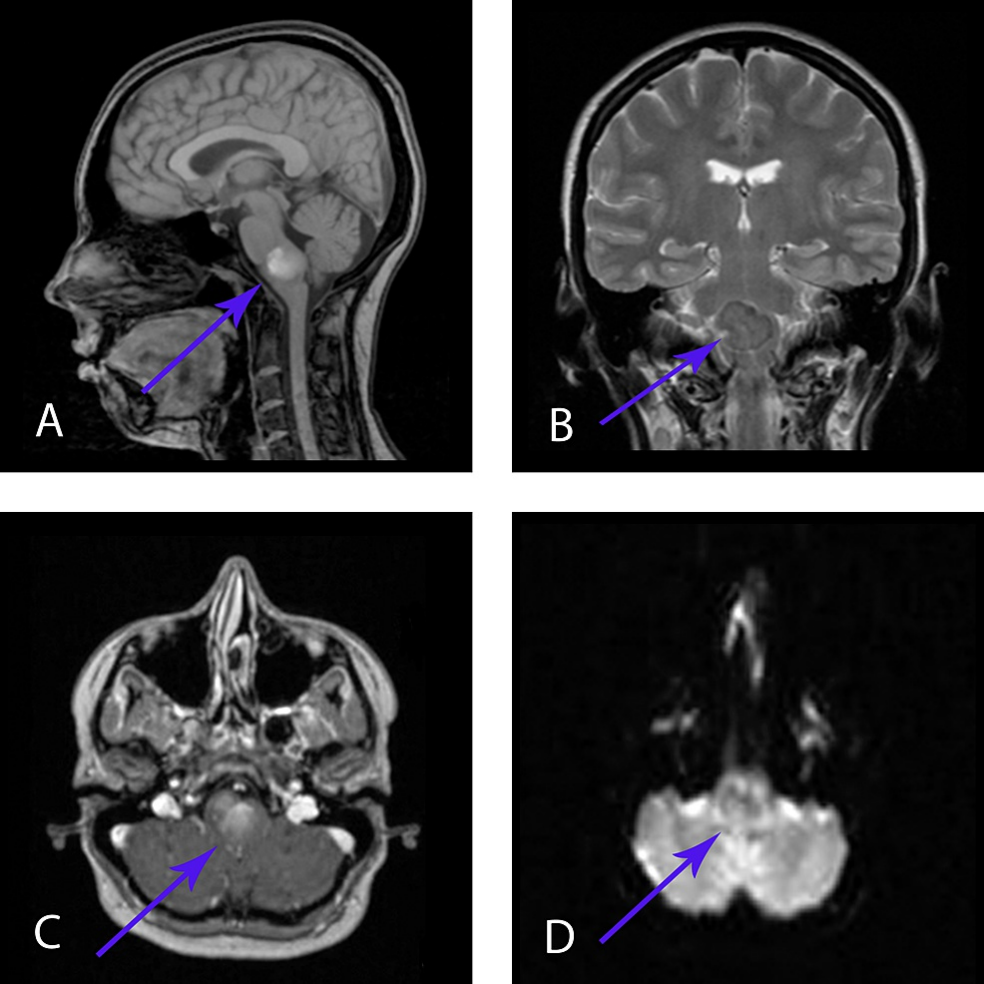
MRI, before the first surgery A – MRI Т1 sagittal view; B – MRI Т2 frontal view; C – MRI T1 + contrast, axial view; D - MRI diffusion-weighted imaging (DWI)
